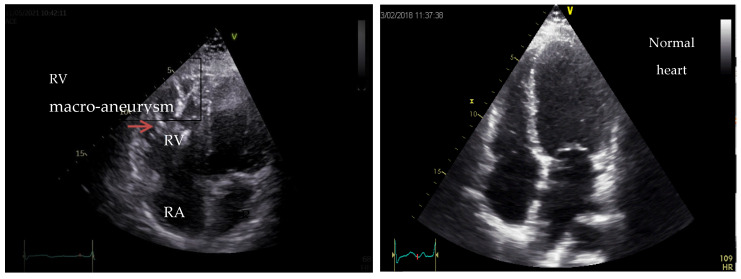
Two-dimensional echocardiography, apical four-chamber view showing right ventricular (RV) dilation with multiple macro-aneurysms and reduced systolic function versus normal heart to the right side of the image.
